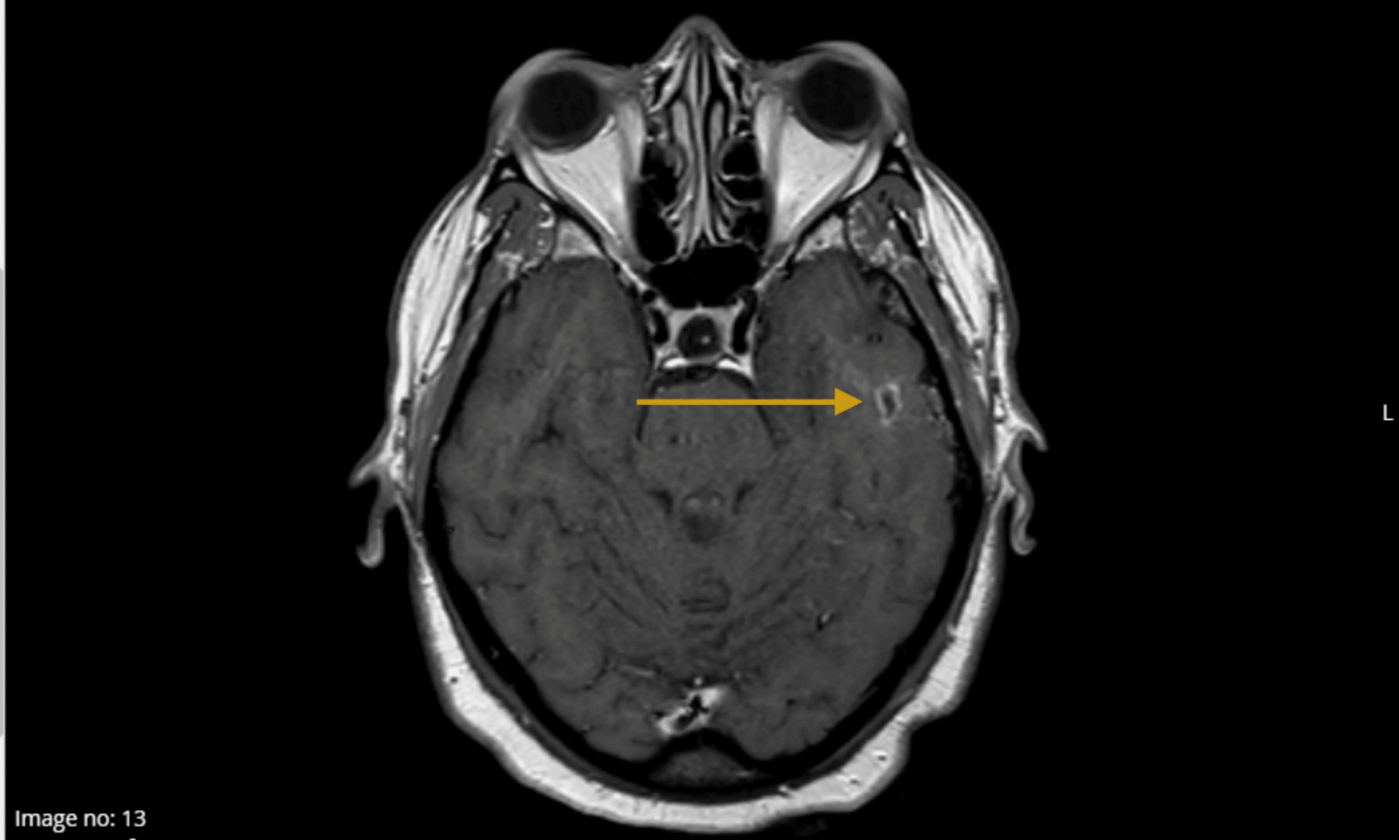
MRI of the brain with contrast MRI brain with contrast (coronal view) demonstrating a small peripherally enhancing fluid collection in the left temporal lobe (measuring 9 × 5 × 7 mm), consistent with a temporal lobe abscess.
